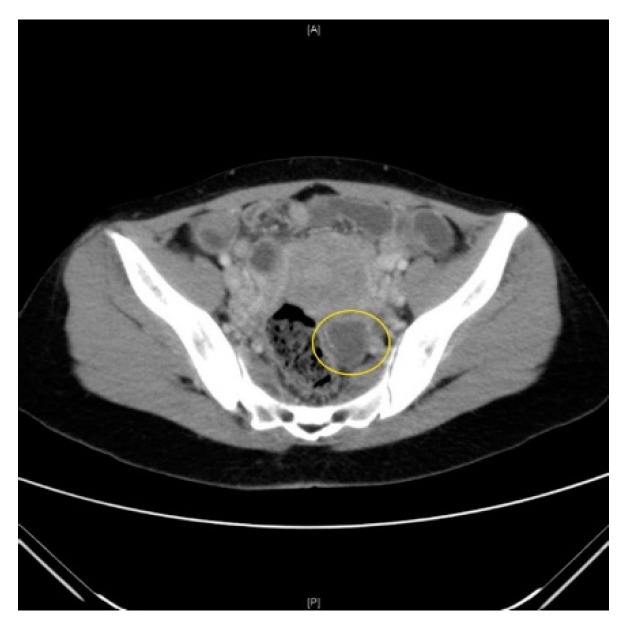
Computed tomography (CT) scan of the abdomen and pelvis with a left partially rim enhancing structure 2 × 2 cm suggestive of a ruptured corpus luteal cyst.
